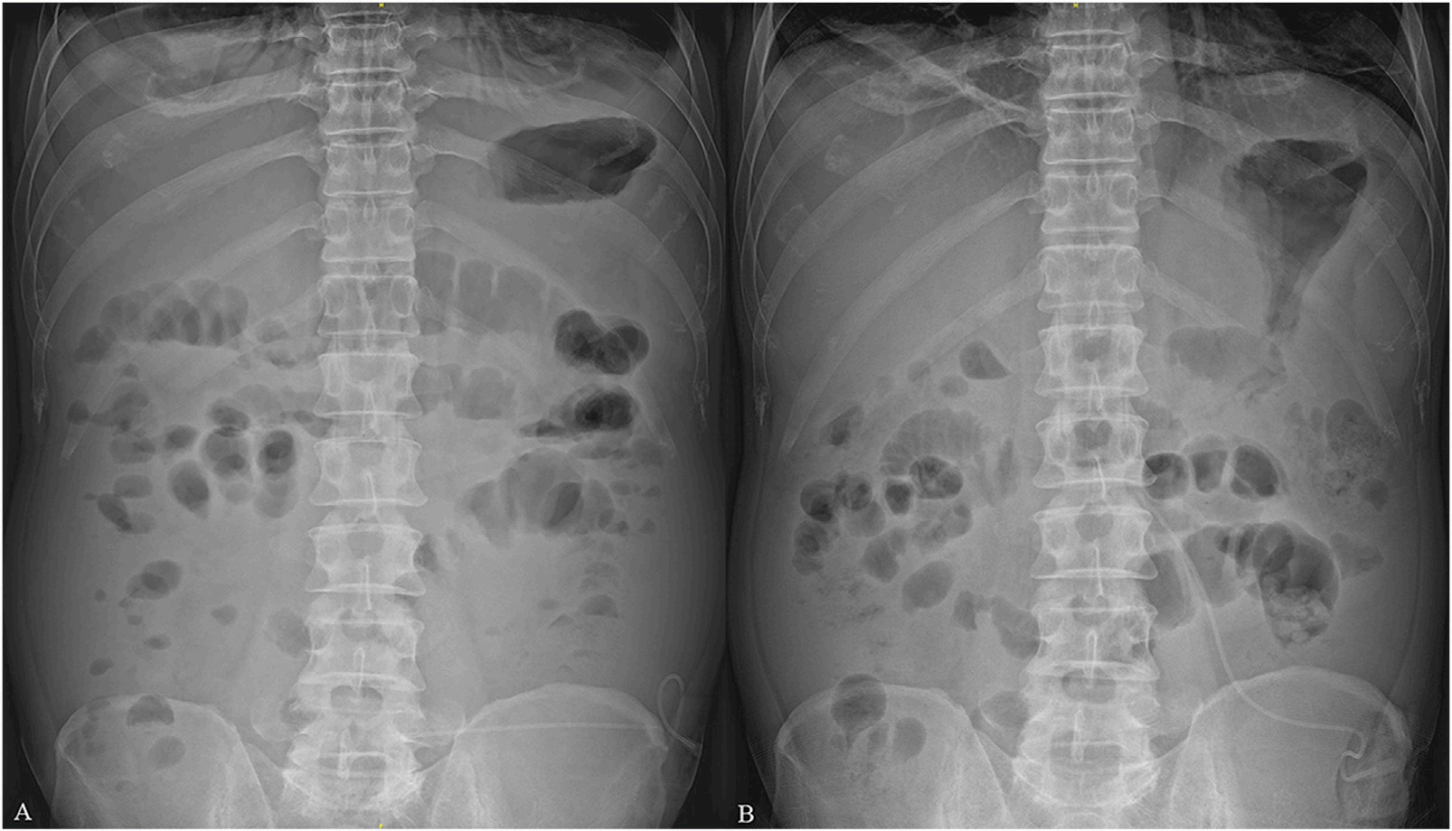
Plain abdominal radiographs of the patient. (A) indicates that the patient exhibited signs of intestinal obstruction on the fifth day after admission, including flatulence and air fluid levels, on plain abdominal radiograph after receiving transdermal fentanyl (TDF). (B) indicates that the patient’s air liquid level disappeared, and the signs of intestinal obstruction improved considerably after TDF was replaced with oral morphine on the 11th day after admission.
